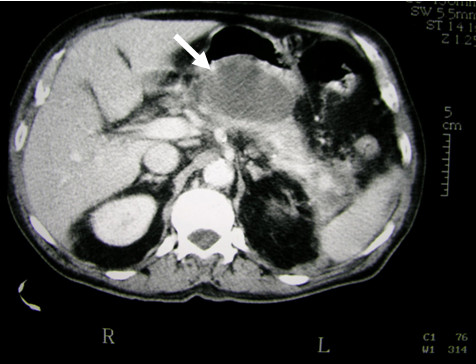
Computed tomographic abdominal scan (CT) showing necrosis and presence of liquid in peripancreatic cavity.
